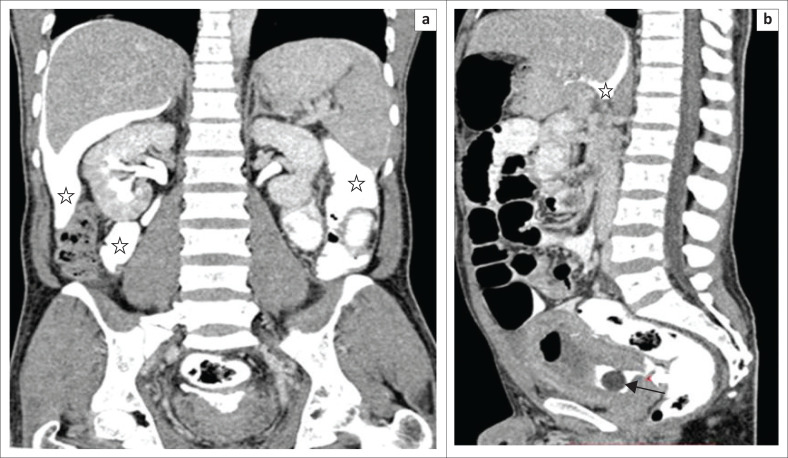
(a, b) Multidetector computed tomography coronal and sagittal reformatted images indicate rupture of the urinary bladder (arrow) with contrast material leaking into the peritoneal cavity, surrounding the bowel loops, along the paracolic gutters and reaching up to the subphrenic space (asterisks). The patient also had localised urethral injury (not shown in this image), for which he underwent MDCTU.
